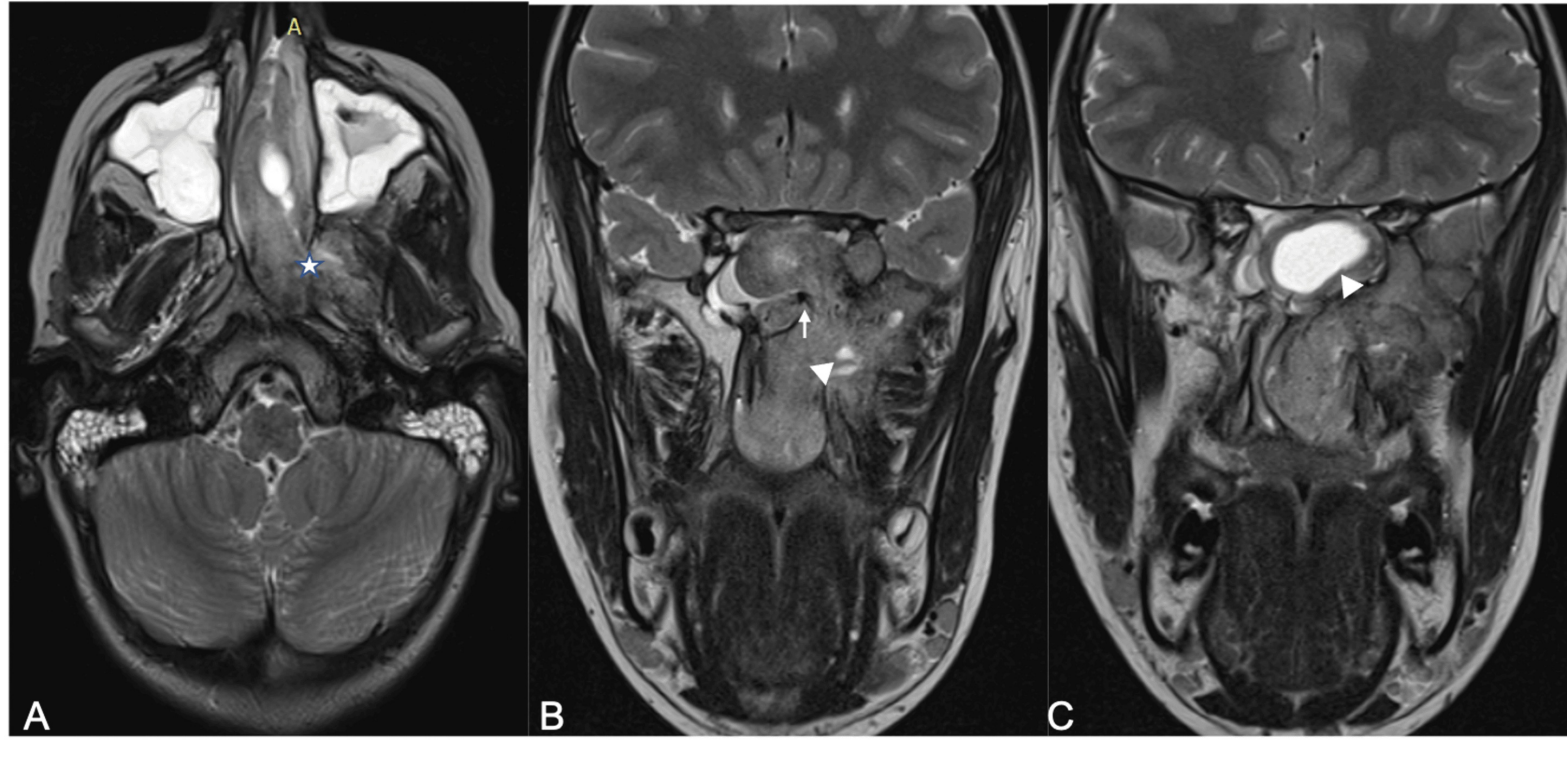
MRI findings of juvenile nasopharyngeal angiofibroma (JNA). Axial (A) and coronal (B, C) T2-weighted images. JNA appears as a mass typically arising from the sphenopalatine foramen, which appears enlarged (asterisk). It classically shows intermediate-to-high heterogeneous signal with serpentine flow voids (arrow) and degenerative cystic components (arrow head).
